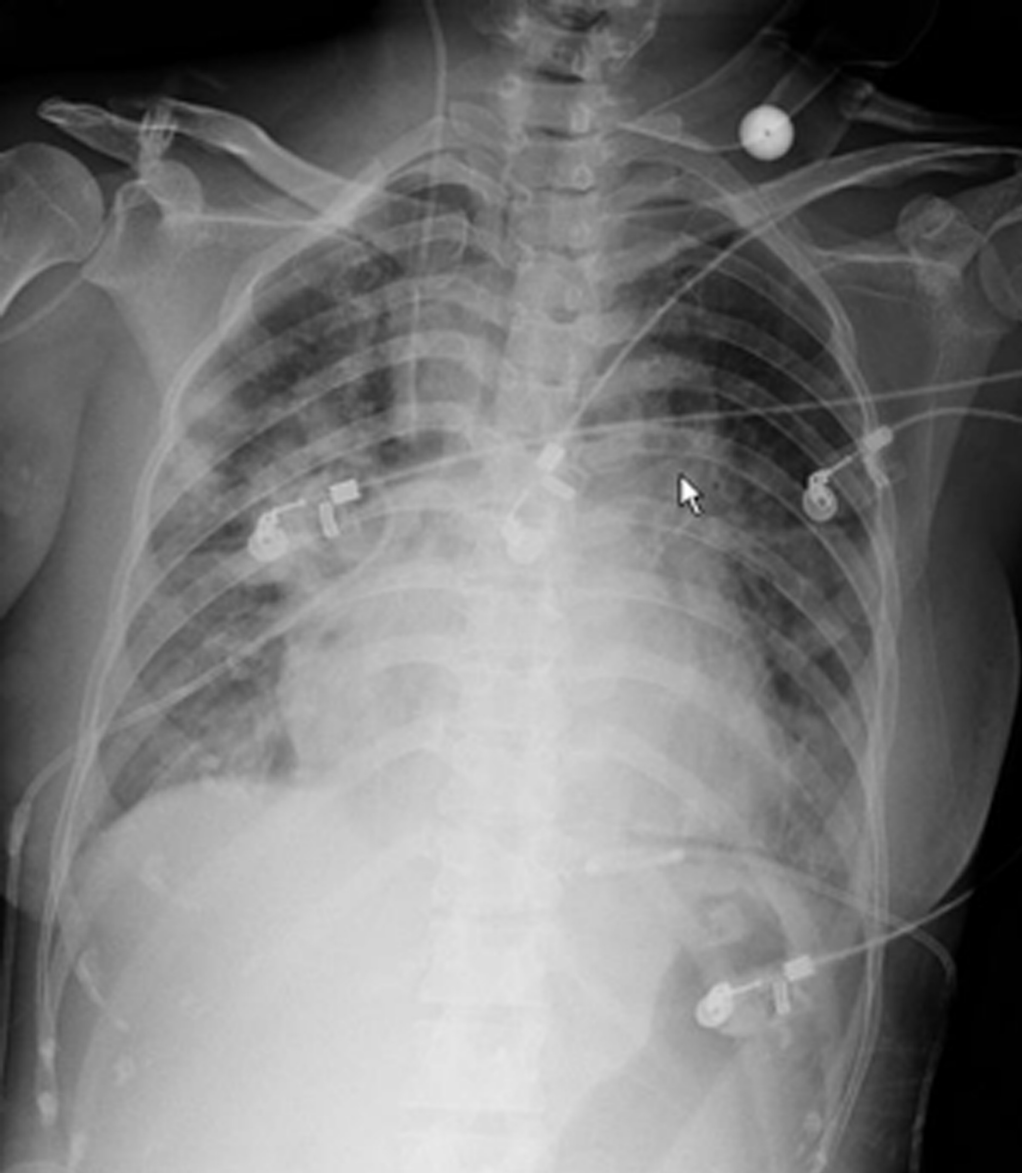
Chest x-ray showing diffuse infiltrates.
